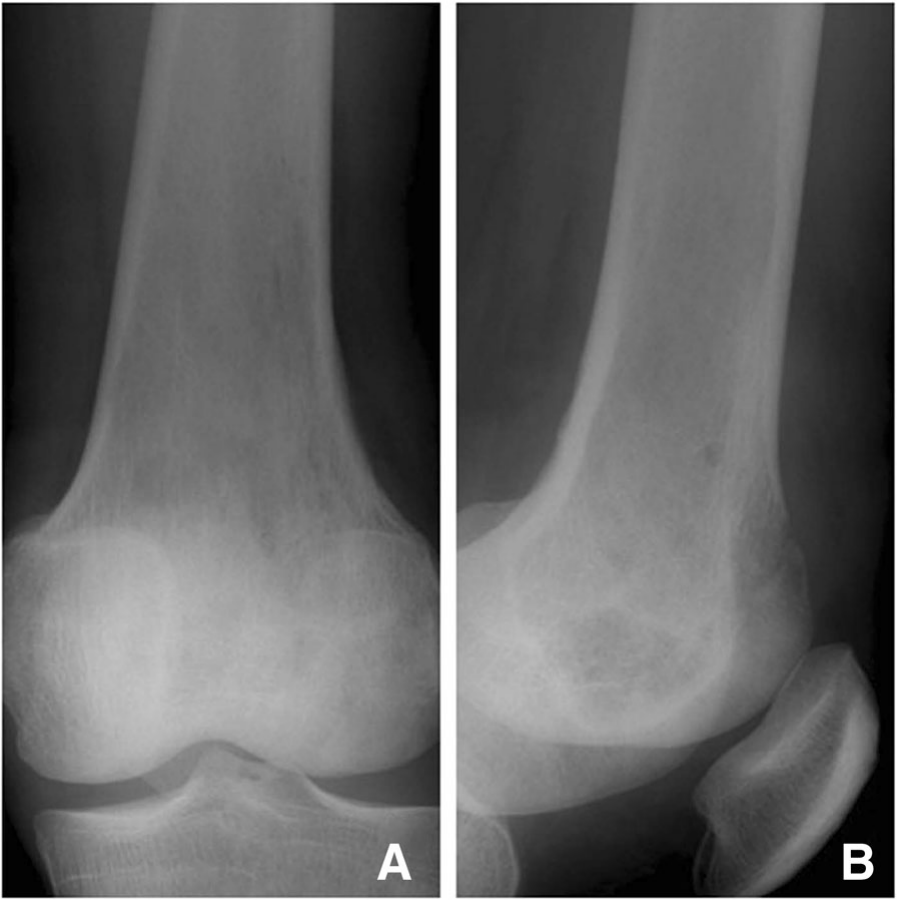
LGMS of the left distal femur. X-ray revealed osteolytic damage and cortex destruction of the left distal femur (a, b)
